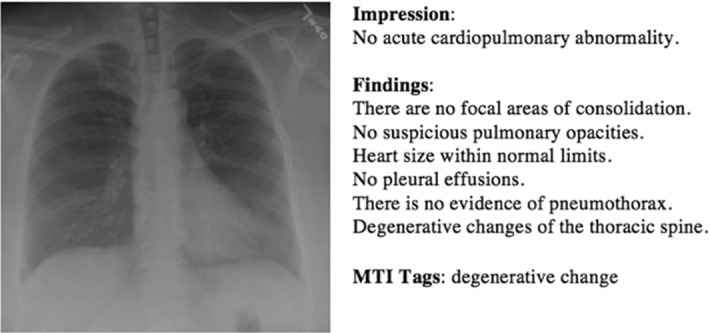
Different sections of the medical reports. Example from the IU X-Ray dataset, retrieved from (Jing et al. 2017)
